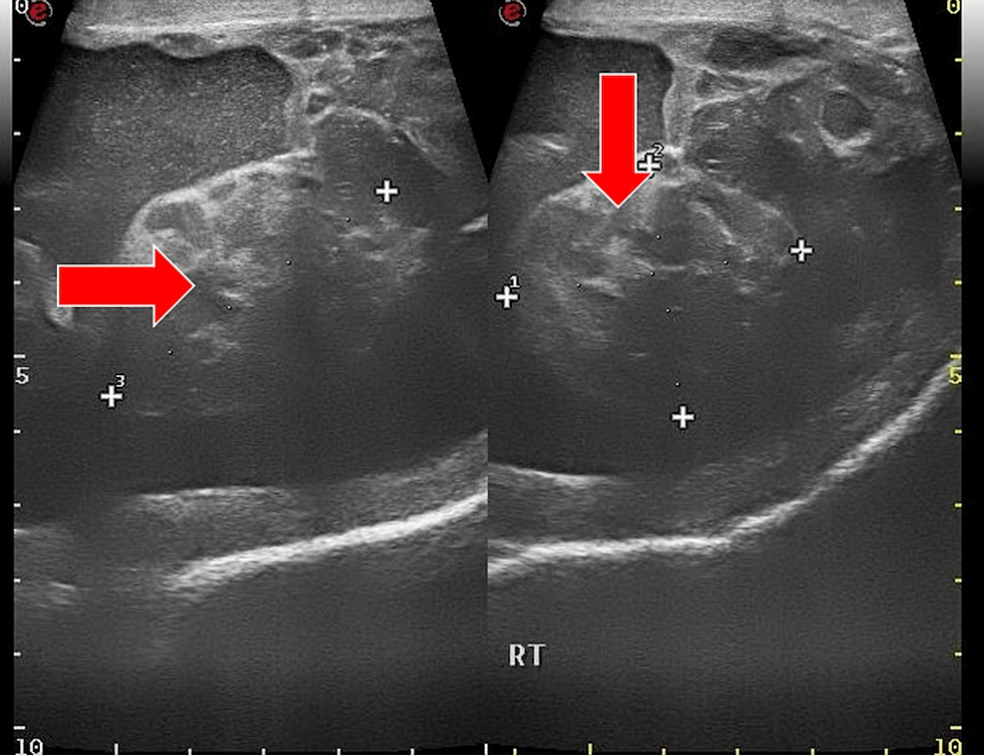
Ultrasound: scrotum - image 2 The image demonstrates heteroechoic mass that is inseparable from the right testis with evidence of a mild amount of echogenic free fluid surrounding the mass lesion
